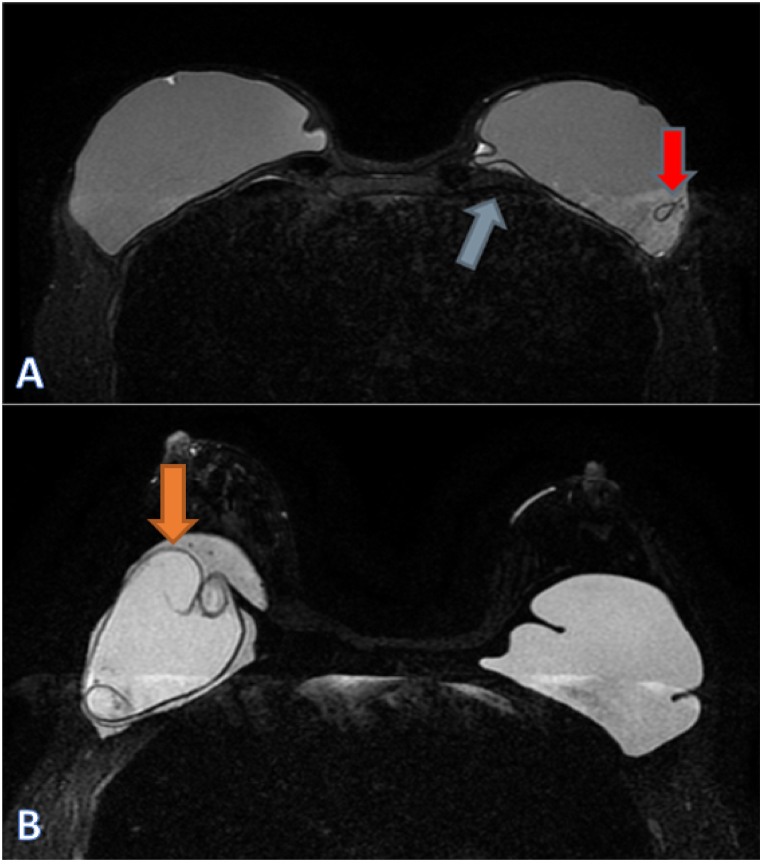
Breast MRI’s with silicone breast implants. A. Blue arrow shows a subscapular line, the red arrow points to a teardrop sign. Both are indicative of an intracapsular implant rupture. B.Axial MRI silicone suppressed image showing an inhomogeneous implant and internal degradation with linguini sign of the left breast (orange arrow), confirming an intracapsular rupture.
